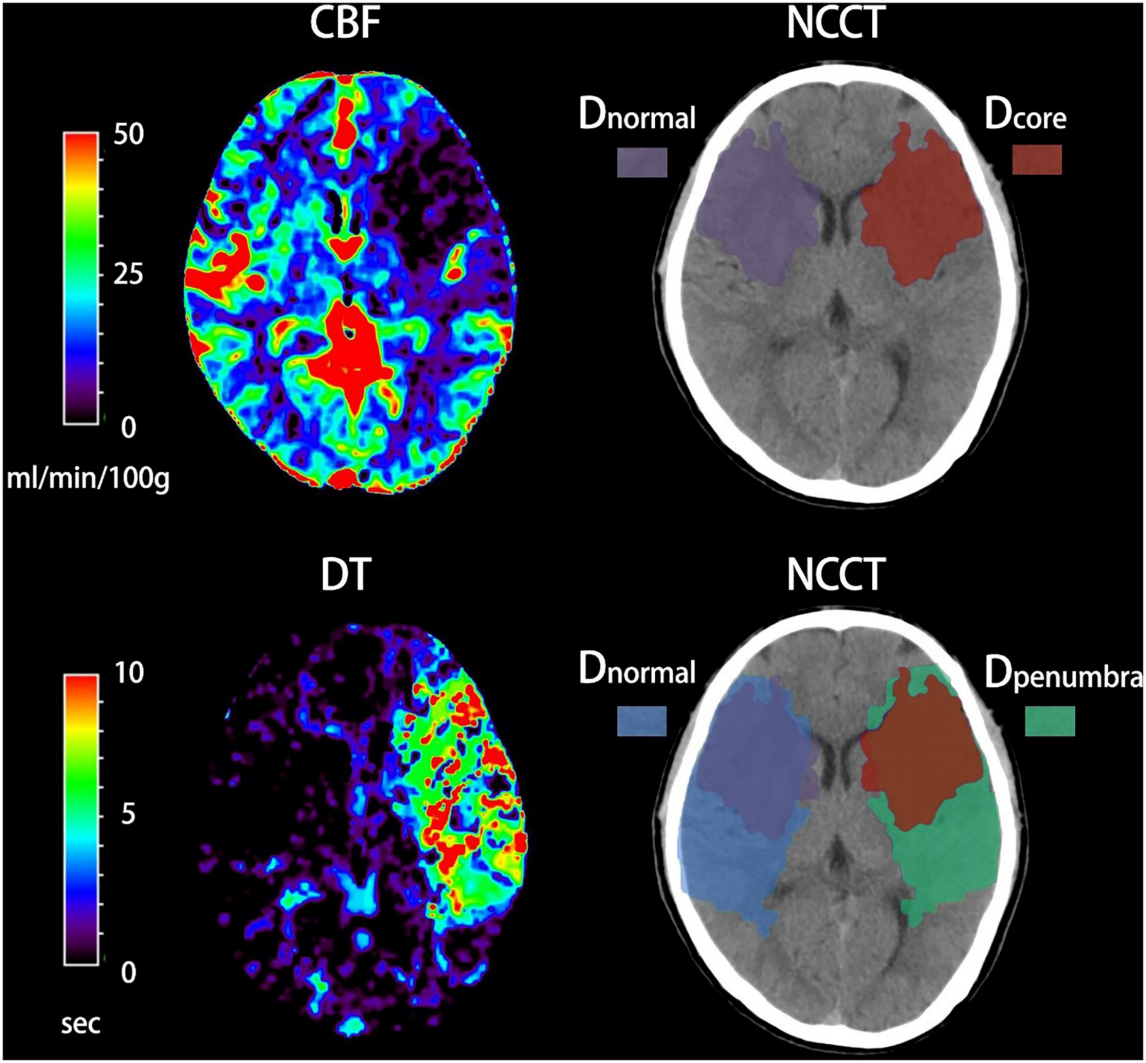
Quantification of net water uptake (NWU) per volume of early infarct in admission non-contrast computed tomography (NCCT). The ischemic core and penumbra were identified by cerebral blood volume (CBV) and delay time (DT) maps based on computed tomography perfusion (CTP). The mean density of early infarct (D_core_ and D_penumbra_) and the normal tissue (D_normal_) derived from a mirrored contralateral region of interest were then calculated.
